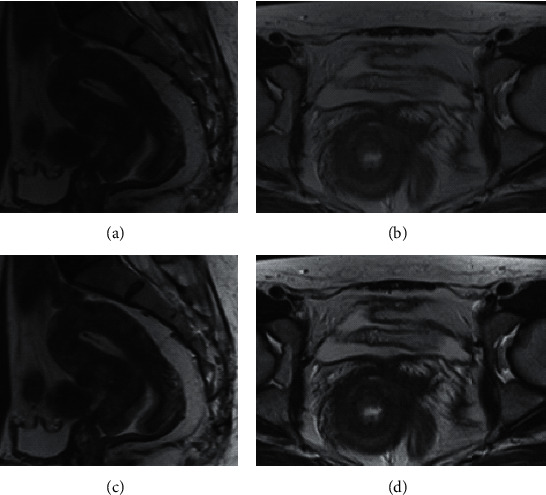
Imaging comparison of two algorithms. A and C are the images of conventional multimodal MRI; B and C are those of multimodal MRI under the alternating direction algorithm.
